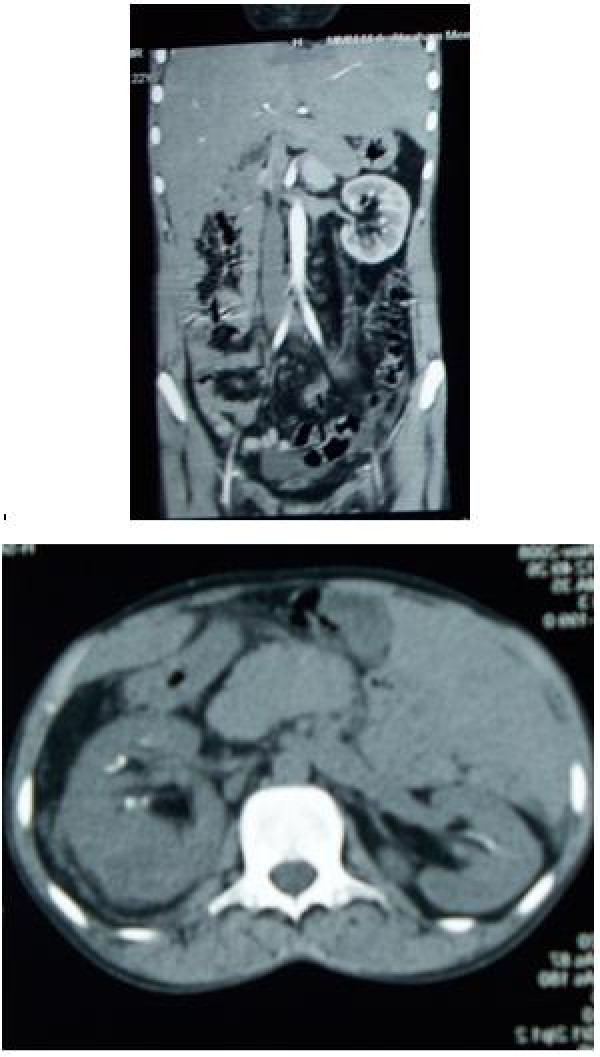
Longitudinal and horizontal abdominal computed tomography images of the affected kidney.
